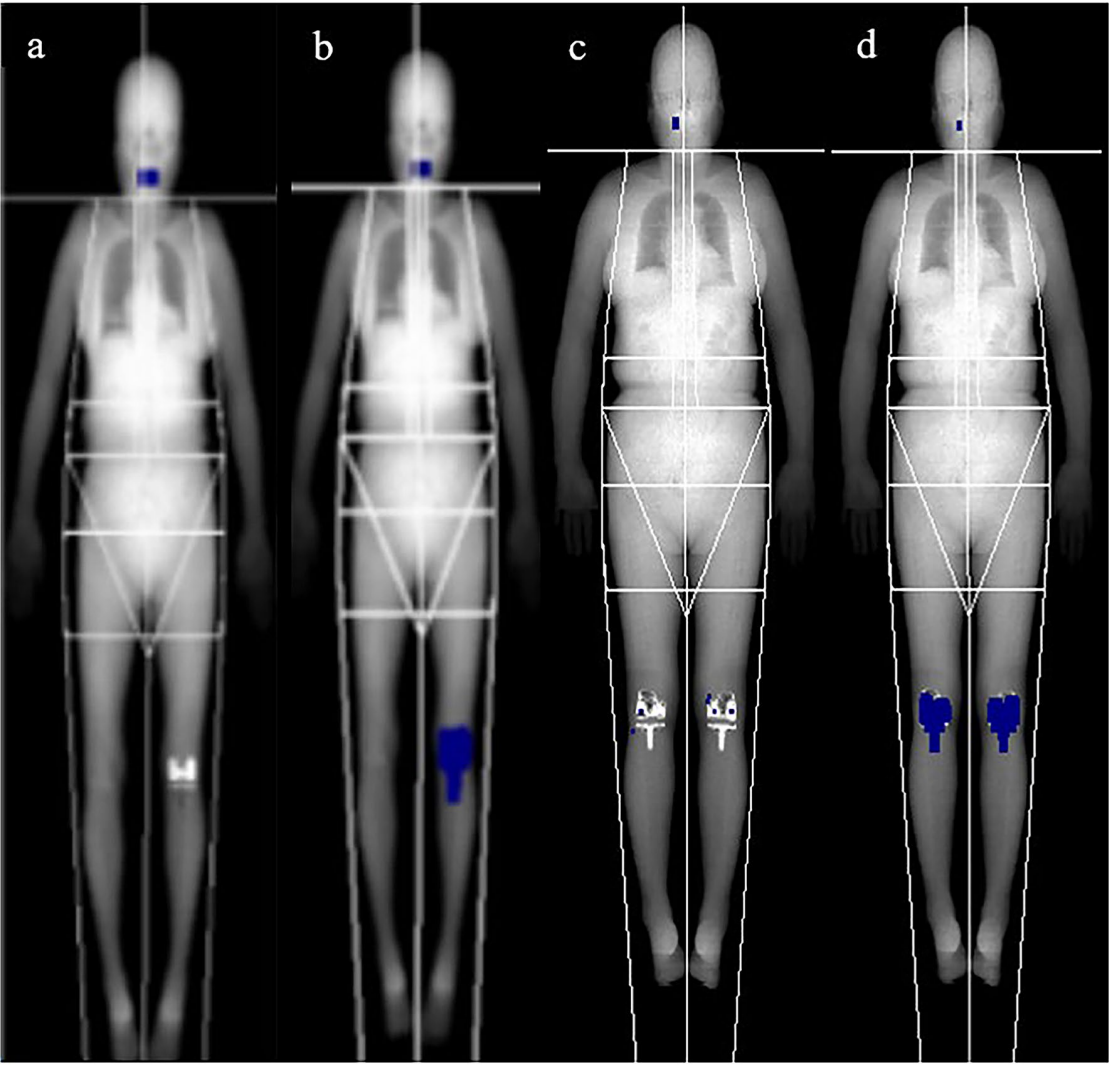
Dual X-ray absorptiometry images with and without automatic metal detection (AMD). (a) Left knee arthroplasty without AMD processing. (b) Left knee arthroplasty with AMD processing. (c) Bilateral knee arthroplasty without AMD processing. (d) Bilateral knee arthroplasty with AMD processing. *Images obtained from the Korean Frailty and Aging Cohort Study.
